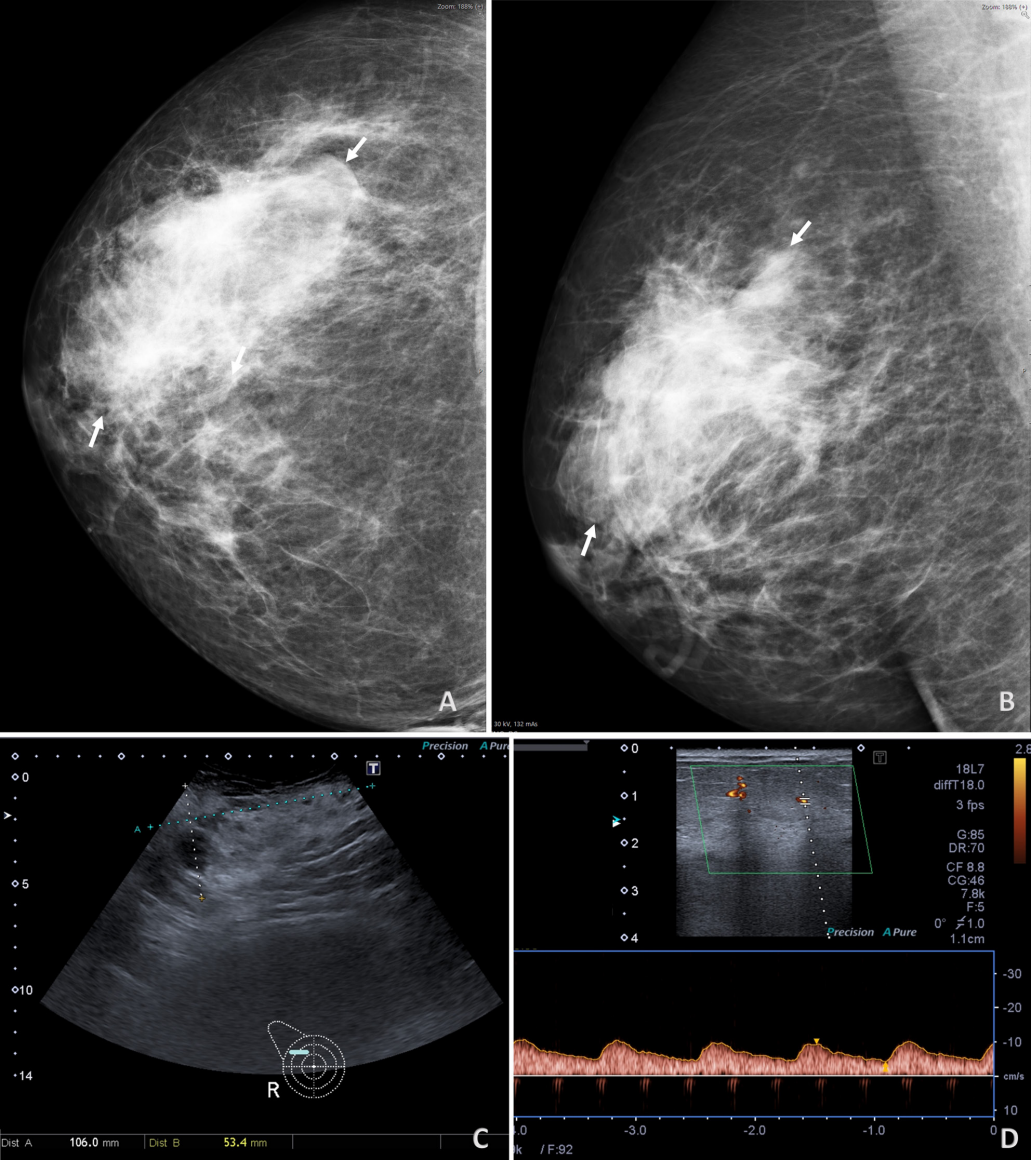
Magnetic resonance imaging (MRI) showed a T2-hyperintense large lobulated mass (arrows, *Figure 2A*), with intra-ductal T1-hyperintense blood products (arrows, *Figure 2B*) and heterogeneous enhancement, mixing areas of intense initial enhancement with washout (*Figure 2C*), typical of malignancy, with others of progressive centripetal filling (*Figure 2E–G*, arrows), suggesting a vascular lesion. There was neither axillary lymph node enlargement nor skin involvement.
